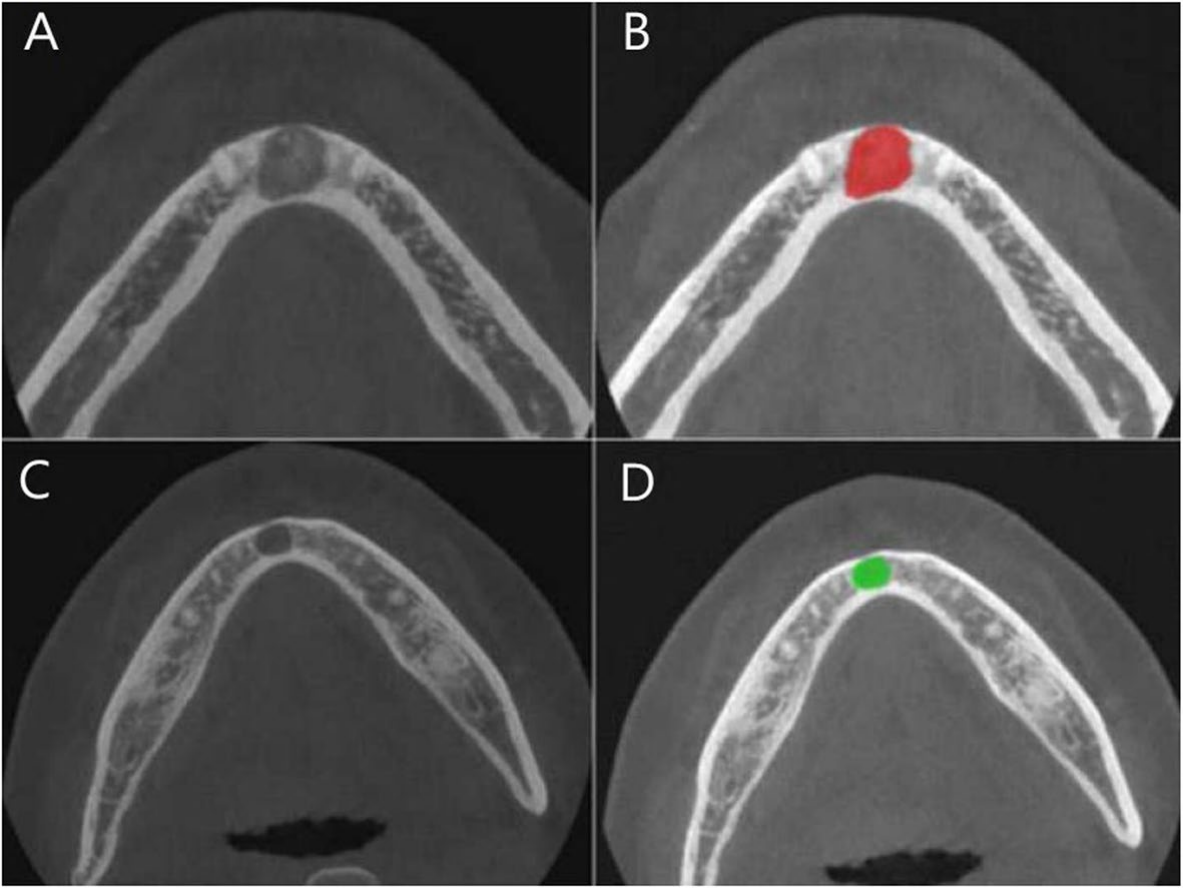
Example of region of interest placement. A 48-year-old woman with a diagnosis of cemento-osseous dysplasia (COD) (A). The cone-beam computed tomography (CBCT) image of the cementoblastic stage showed a radiopaque mass in the radiolucent lesion. Manually polygonal outline of the region of interest (ROI) along the outer side of the COD, which is indicated by the red area (B). A 52-year-old man with a diagnosis of periapical cyst (PC) (C). The CBCT image of the periapical cyst showed a radiolucent lesion with a radiopaque edge. Manually polygonal outline of the ROI along the outer side of the PC, which is indicated by the green area (D)
